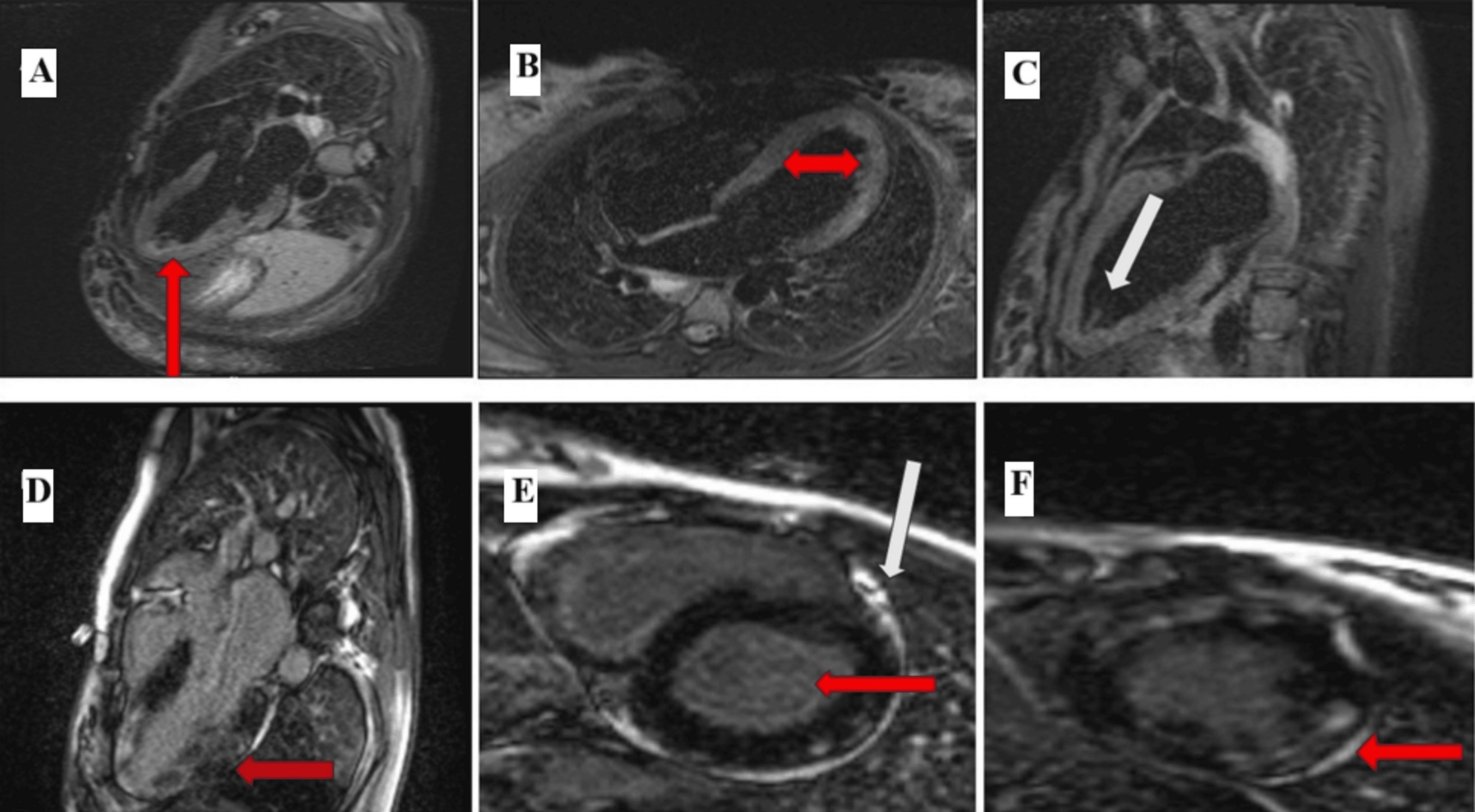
Cardiac magnetic resonance imaging (A-C) T2-weighted images; long axis with three-chamber, four-chamber, and two-chamber views showing evidence of significant active inflammation/edema involving predominantly the lateral wall and all mid distal segments, with the highest values toward the apex. (D) Long-axis view T2-weighted image showing left ventricular inflow-outflow plane. Arrow indicates a patchy intramyocardial gadolinium uptake pattern in the lateral wall and all mid-distal segments with fibrosis consistent with significant active myocarditis. (E,F) Short-axis view with late gadolinium enhancement from subepicardial to mid-wall in the basal distal lateral, mid-distal septal, and other apical segments, becoming near transmural in the distal segments
